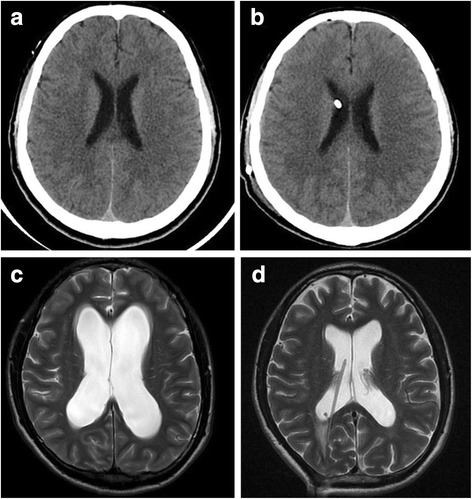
a and b Head CT of a 40-year-old VPSWOV patient before and after operation. a Pre-operative CT shows no dilation of the cerebral ventricles (b) postoperative CT shows no dilation of the cerebral ventricles. c and d Head MR of a 28-year-old VPSV patient before and after operation. a Pre-operative axial T2-weighted image shows dilation of the cerebral ventricles (b) postoperative T2-weighted shows narrowing of the cerebral ventricles
